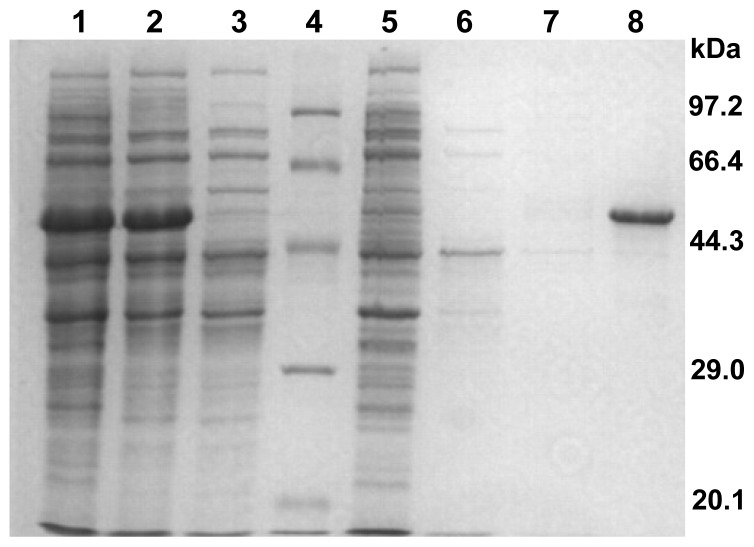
SDS-PAGE gel electrophoresis of the purified CbPelA protein. Lane 1: supernatant of the cell lysate treated by ultrasound; Lane 2: supernatant of the product after thermo-treatment; Lane 3: elution product following filtering through a Ni–NTA agarose column; Lane 4: protein marker; Lanes 5–8: samples eluted with 0, 20, 50, or 100 mM imidazole, respectively. Molecular mass is indicated at the right of the figure.
